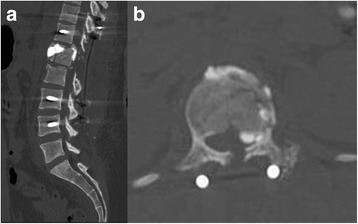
Cement leakage into the spinal canal after vertebroplasty. Vertebroplasty without balloon inflation causes cement leakage through the cortical disruption as detected on postoperative computed tomography scanning (a, b)
